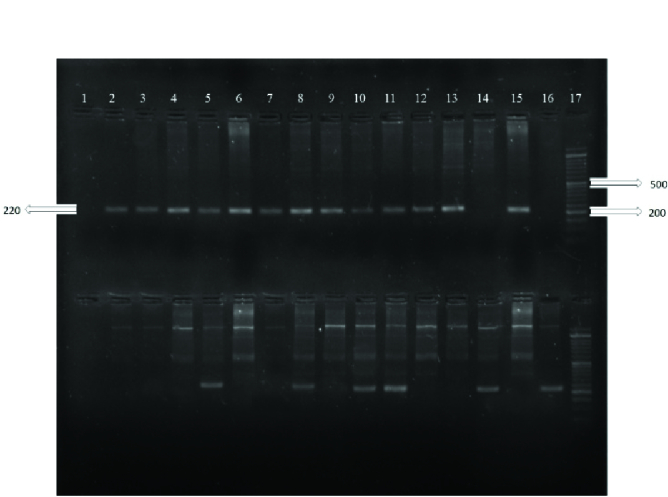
ARMS-PCR products of rs1048943. The top bands show wild alleles and the down bands show mutant alleles for each sample: Number 1 is negative control; 2, 3, 4, 6, 7, 9, 12, 13 and 15 are wild homozygotes (TT), 5, 8, 10 and 11 are heterozygotes (CT), 14 and 16 are mutant homozygotes (CC), and 17 is 50 bp DNA ladder.
